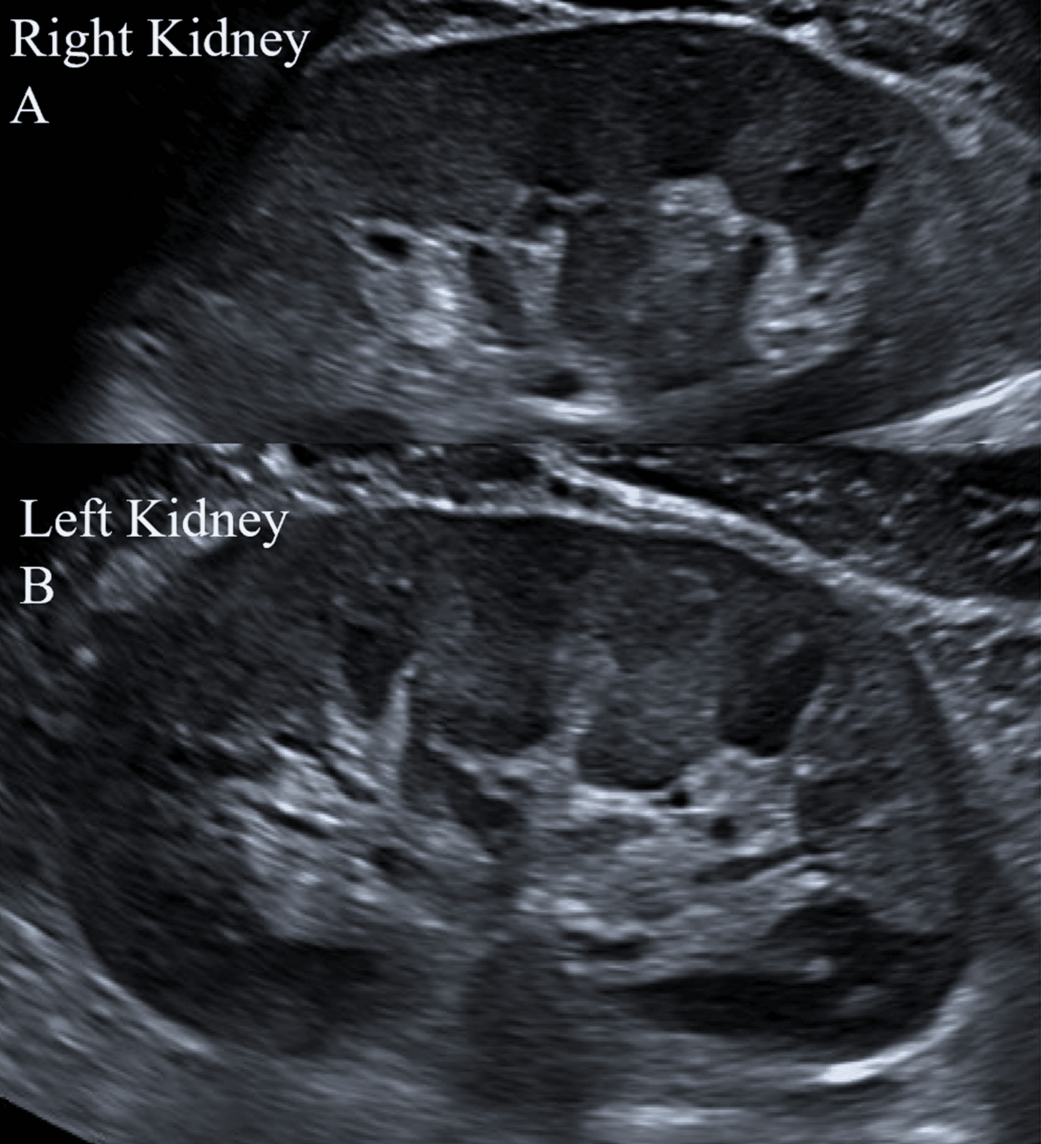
Renal tract ultrasound revealed no structural abnormalities in the right (A) and left (B) kidneys.
